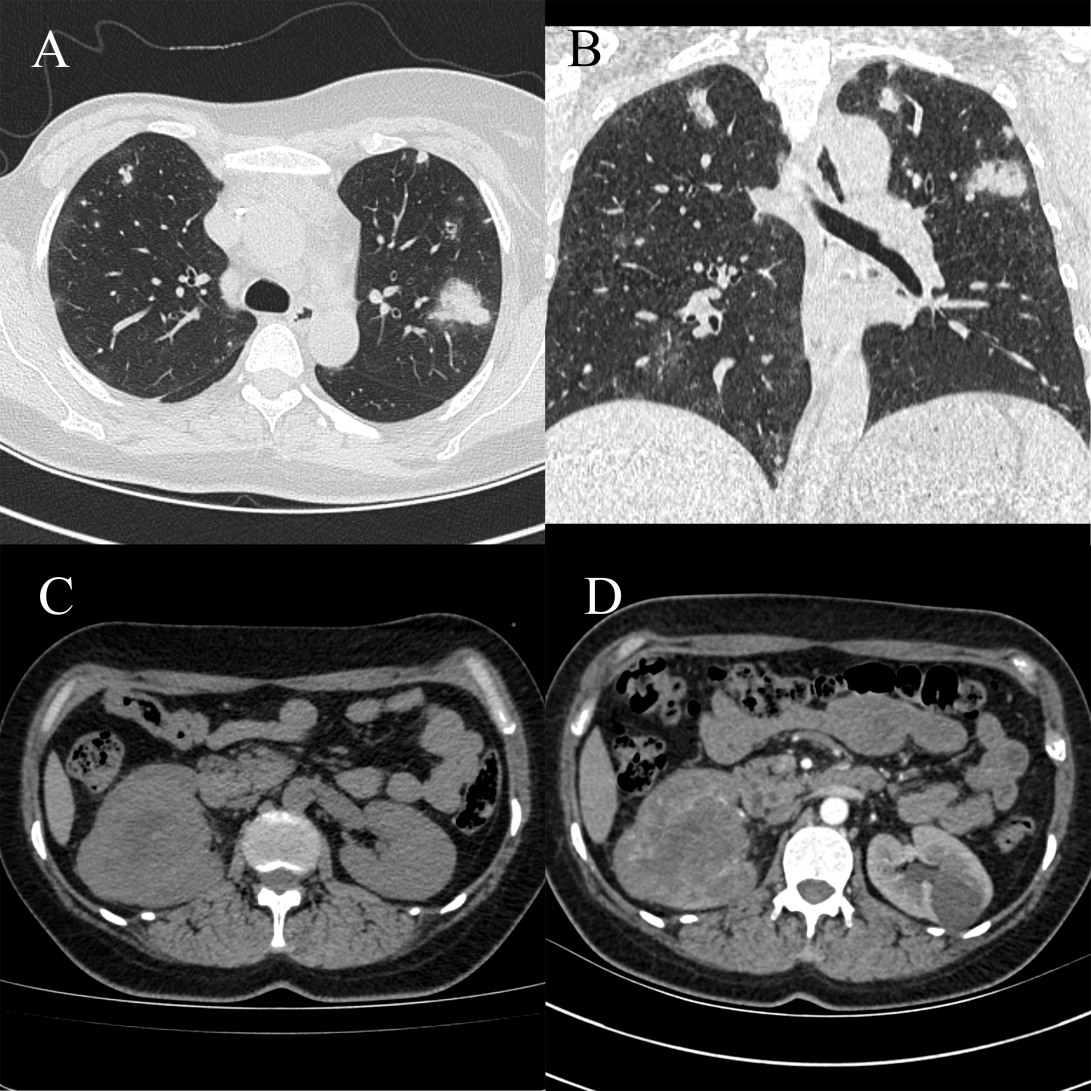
Computed tomography (CT) scan showing an increase in the number and size of lung lesions compared to previous results, with a slight increase in the right renal sinus mass. (A) Chest CT scan, horizontal position. (B) Chest CT scan, coronal position. (C) Abdominal CT scan, horizontal position. (D) Abdominal CT scan, arterial phase, horizontal position.
